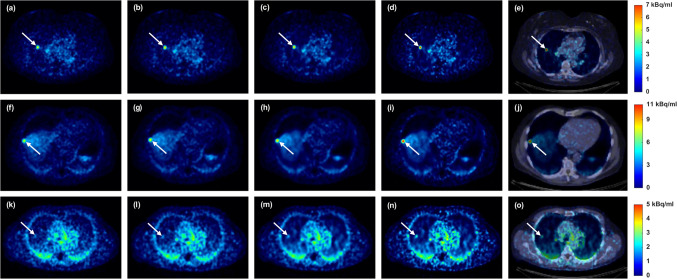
Image comparison of artificial lung lesions. From left to right, the columns show non-PRC, tissue-independent PRC, TDSV PRC with 6.0 mm FWHM filter, TDSV PRC with 4.0 mm FWHM filter, and PET/CT fusion for the TDSV PRC with 4.0 mm FWHM filter. Lung_1_: (a)-(e). Lung_2_: (f)-(j). Lung_3_: (k)-(o)
